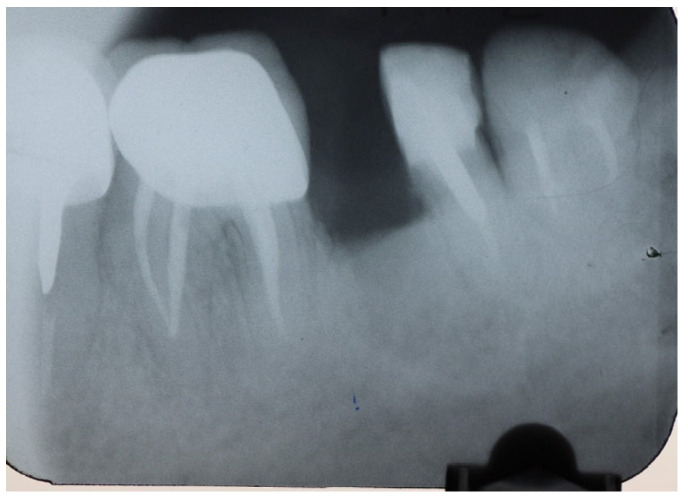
Postsurgical X-ray following hemisection of tooth #37.
